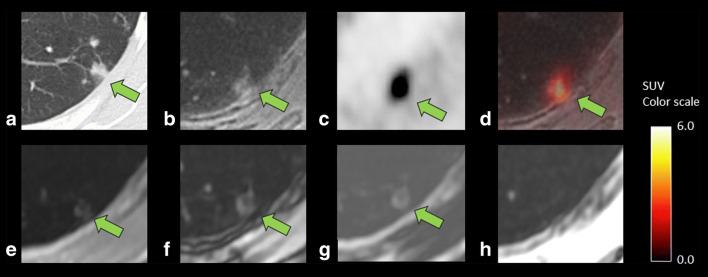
A case showing lung inflammatory changes in a 75-year-old woman after surgery of pancreatic cancer. CT (a) showed a 7.9-mm-sized lesion with a linear margin and a component of ground-glass opacity, which were concordant findings with an inflammatory process. ZTE (b) can clearly reveal these CT characteristics for the margin and the opacity, leading to correct diagnosis of active inflammatory lesions by combined findings of the high FDG uptake (SUVmax 6.9) on PET (c) and fused images (d). Dixon sequence (e, in phase; f, out of phase; g, fat; and h, water image, respectively), however, failed to depict those characteristics of the component of ground-glass opacity and simply showed a nodule-like lesion in comparison with ZTE, resulting in incorrect diagnosis of malignant nodule
